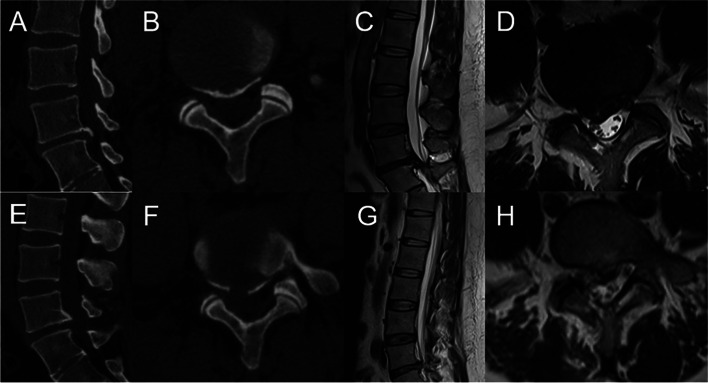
Pre- and post-operative MRI and CT (A-H). A-D Preoperative CT and MRI showed lumbar disc herniation combined with calcification. E–H Postoperative CT and MRI revealed the loosened nerve root after the calcified disc was removed by PETD
